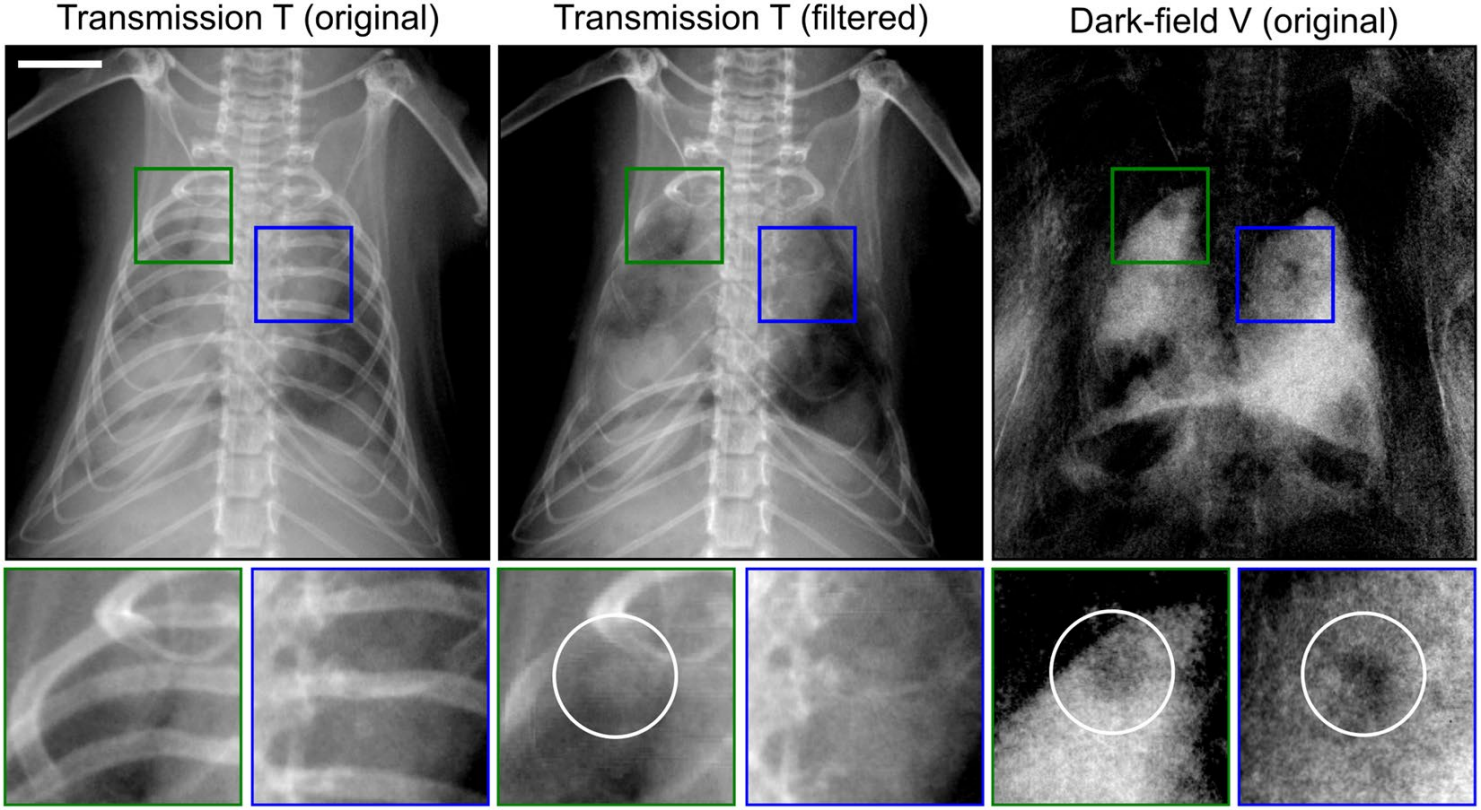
Outlook: initial comparison of ribcage-corrected transmission versus dark-field radiography and impact on tumor detection quality. In the left upper lung (green box) a small nodule is superimposed by multiple ribs and is visible only after ribcage removal, as indicated by a white circle in the filtered transmission radiogram. In case of a small lesion in the upper right (blue box), however, the tumor provides insufficient contrast with respect to healthy tissue, rather then being superimposed by bones. Consequently the suppression algorithm is not altering the detectability of the tumor. Note that both tumors are easily visible in the dark-field channel (white circles), contingent on a strongly enhanced sensitivity towards distortions in the micromorphological lung pattern caused by cancerous tissue. The scale bar corresponds to 5 mm.
